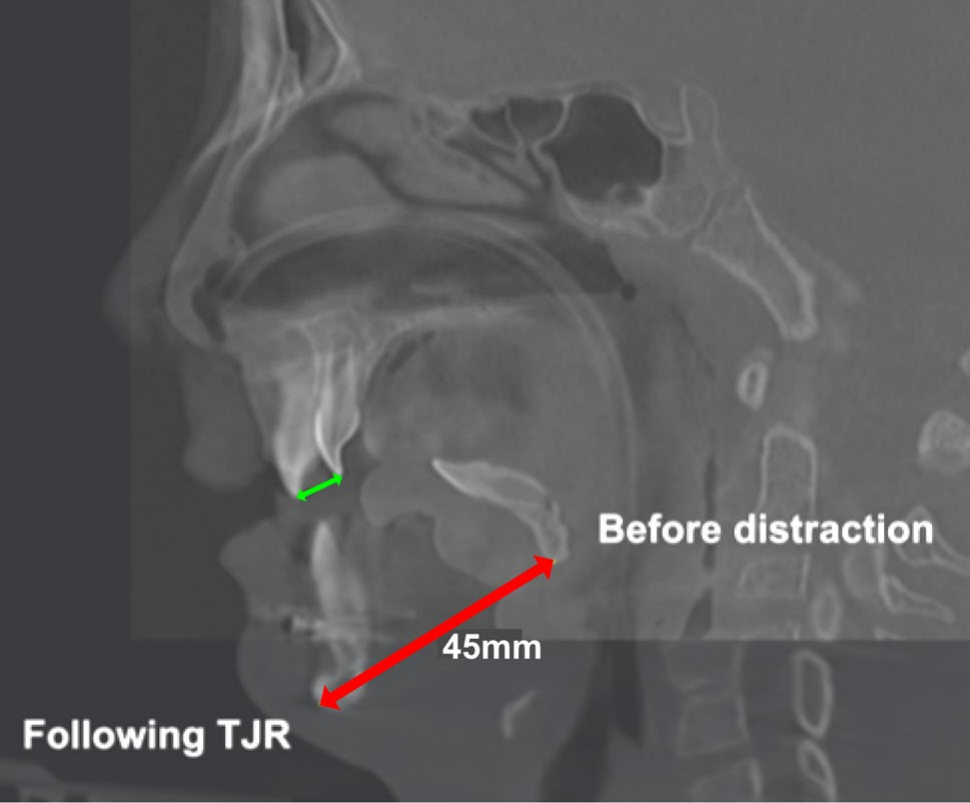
Case 3—Fusion of initial/pre-distraction (surgery prior to TJR) CBCT-scan and postoperative/post-TJR CBCT-scan. Patient suffered from severe bilateral mandibular hypoplasia with permanent tracheotomy and being unable to eat. Red arrow indicates large distance mandibular movement between initial situation and situation after TJR (45 mm). Movement between post-distraction and post-TJR situation was 20.1 mm (Table 2). Green arrow indicates maxillary growth between both CBCT-scans
